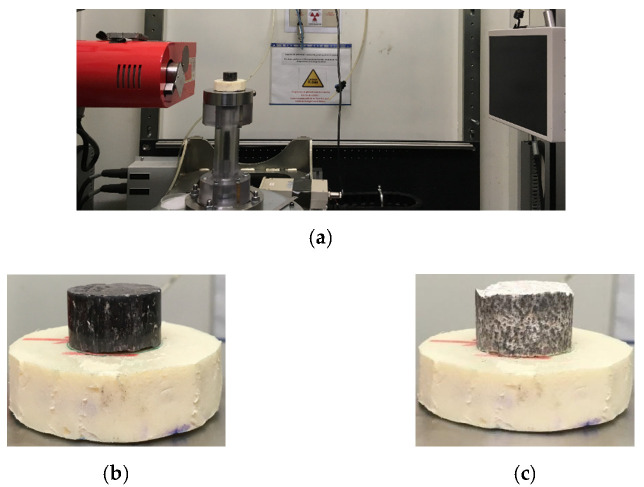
X-ray tomographic system for geotechnical studies: (a) The device at Grenoble has a maximum resolution of 5 µm; (b,c) calcareous and siliceous sand samples respectively, after compression.
